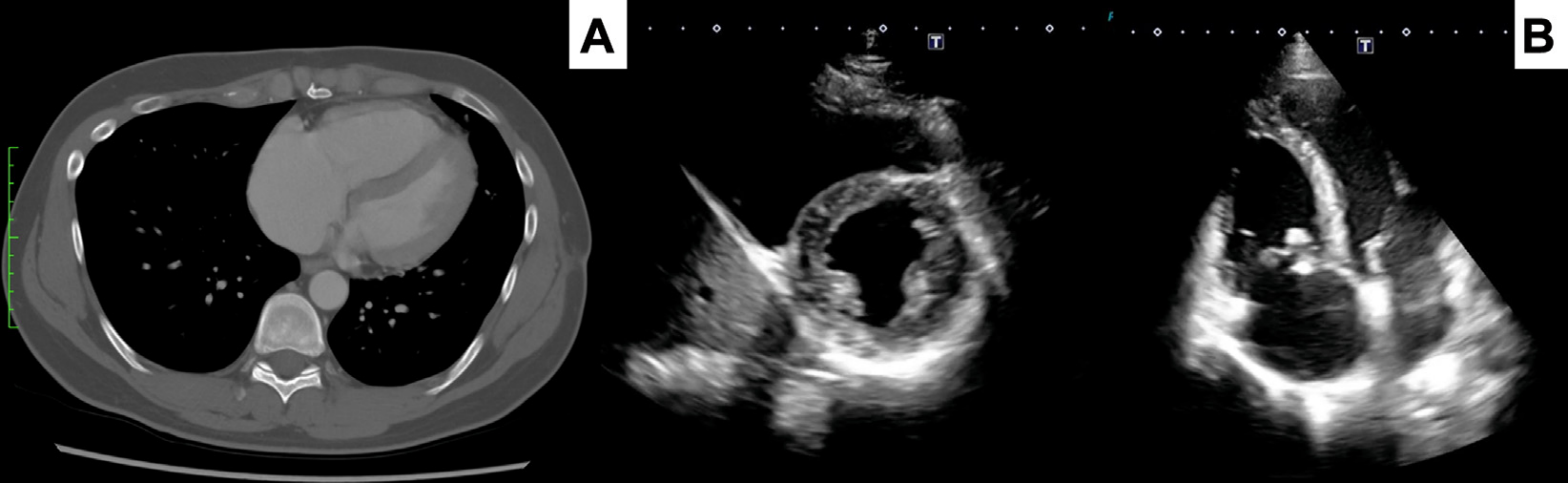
Postoperative Image of the Right Ventricle (A) Postoperative computed tomography findings. (B) Postoperative transthoracic echocardiography showing no residual tumor in the right ventricle.
